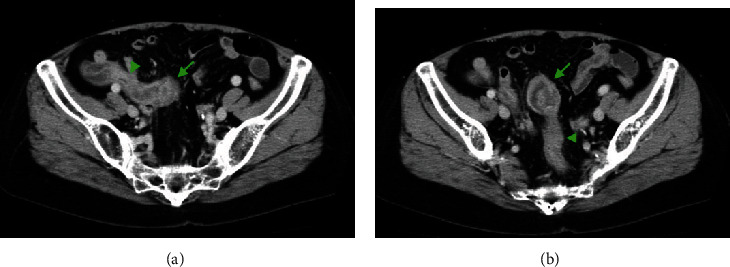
CT findings. (a) An appendiceal mass was seen at the distal part of the appendix. The appendiceal mass and the appendix are indicated by an arrow and an arrowhead, respectively. (b) The appendiceal mass involved the sigmoid colon. The mass and the normal rectosigmoid region are indicated by an arrow and an arrowhead, respectively.
